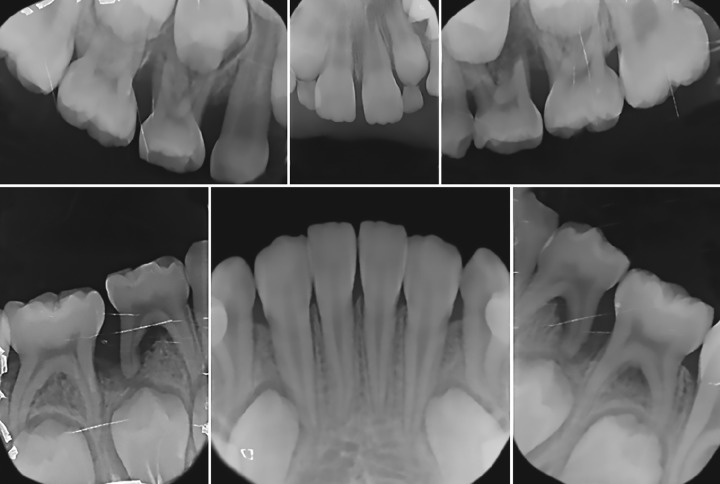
Radiographic situation before therapy of the OS (Periapical radiography)
